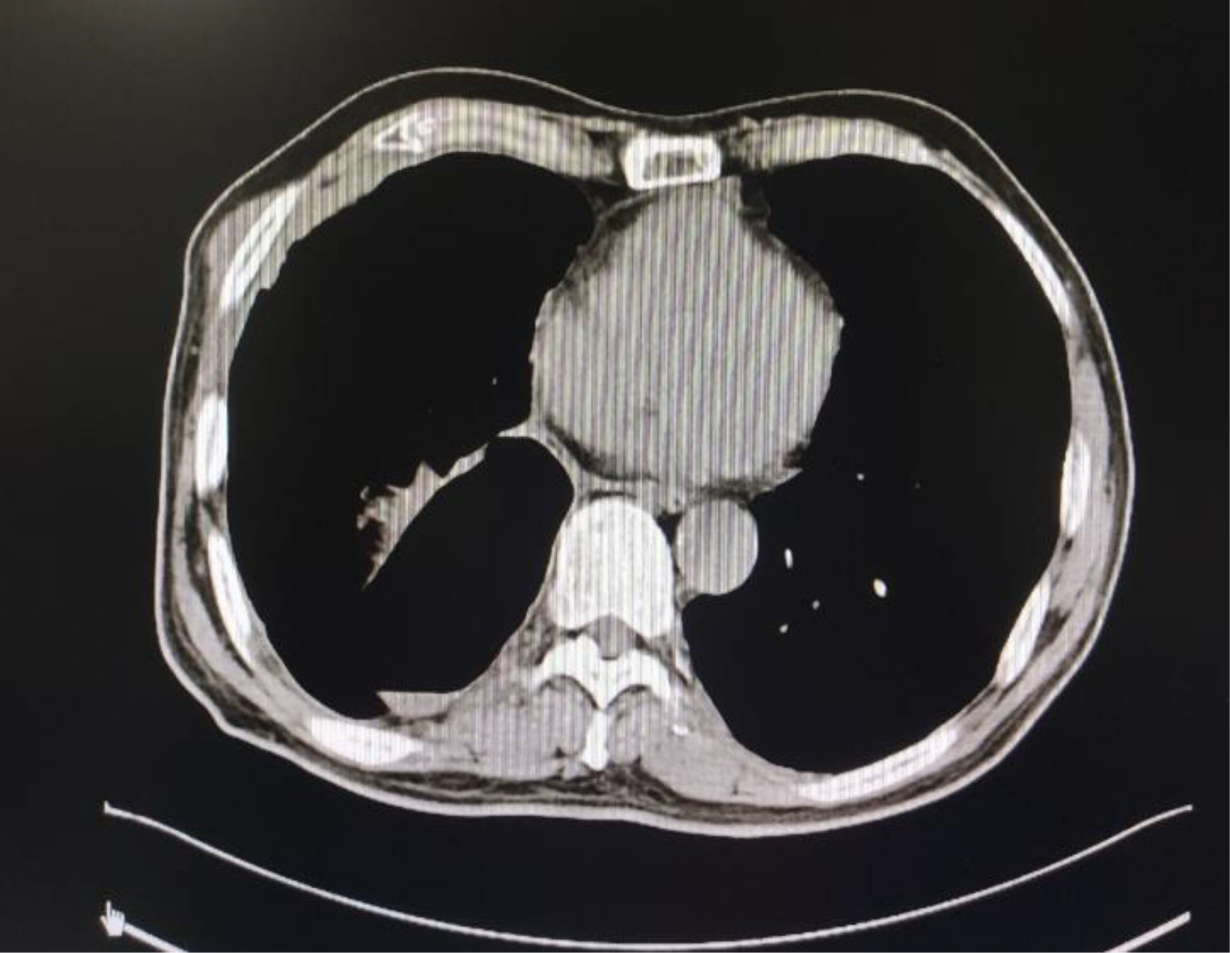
Chest CT after lung surgery and after two cycles of postoperative treatment. The right pleural effusion decreased significantly.
